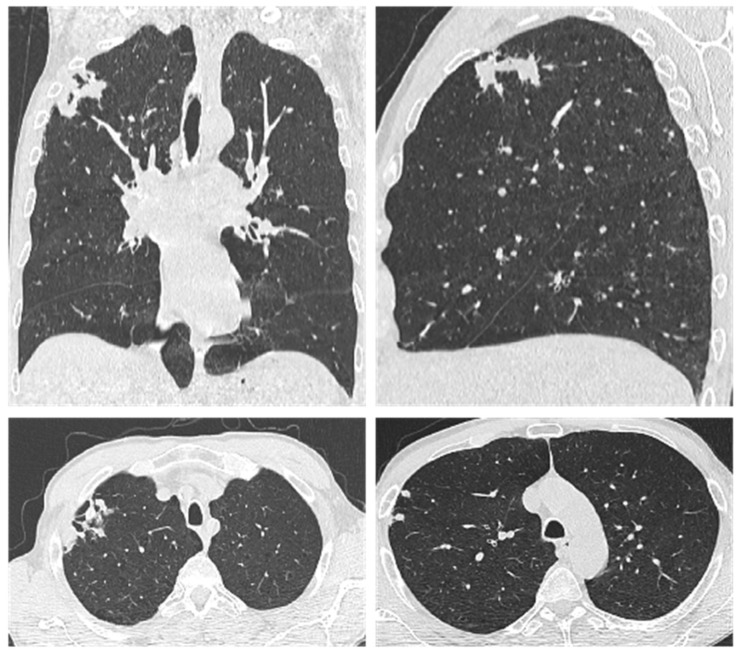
Chest CT from 13 January 2023 showed isolated and confluent nodular and micronodular opacities, some with pleural connection and included microcalcifications, located in the apical and anterior segment of the right upper lobe, bilateral centrilobular pulmonary emphysematous dystrophy, predominantly in the upper lobes.
